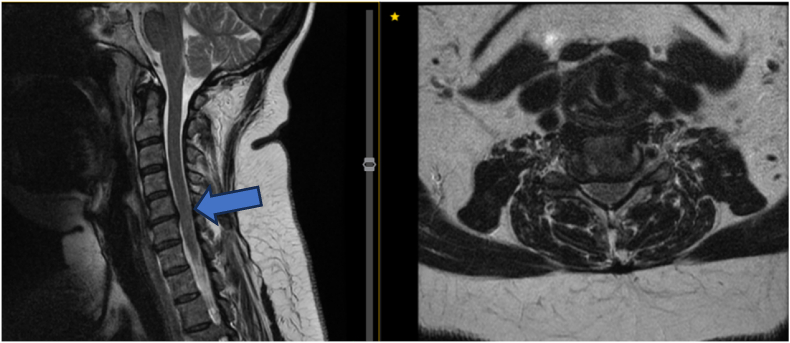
MRI revealed chronic contained C5-6 disc herniation with loss of disc height and bilateral foraminal stenosis.
